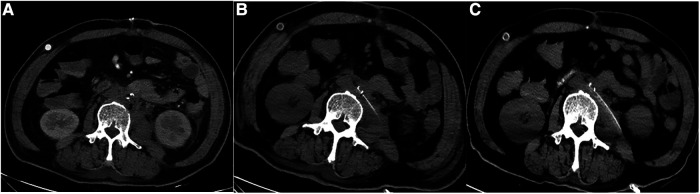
Abdominal CT was repeated at different points after the operation (patient 1). (A)Abdominal aortic CT at 1 week after operation showed that there was still a small amount of gas in the stump. (B) Abdominal aortic CT at 2 weeks after operation showed that the residual air was further reduced. (C) Abdominal aortic CT at 1 month after operation showed that the residual air basically disappeared.
